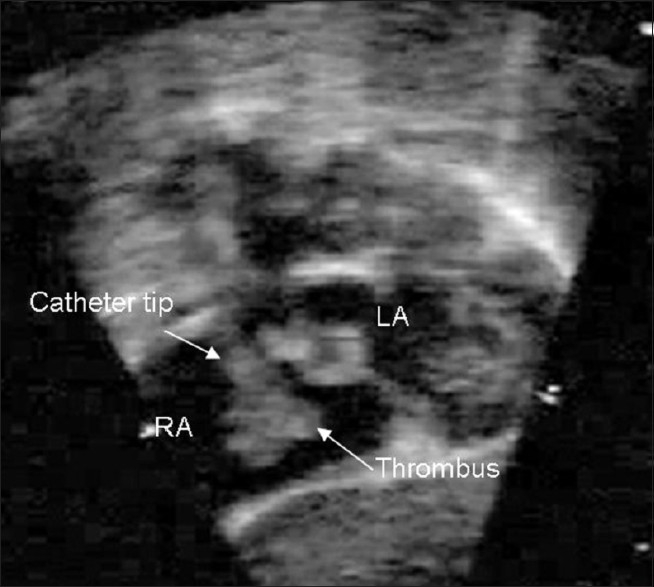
Two-dimensional echocardiography, a subcostal saggital view showing thrombus attached to the catheter tip in the right atrium (RA)
